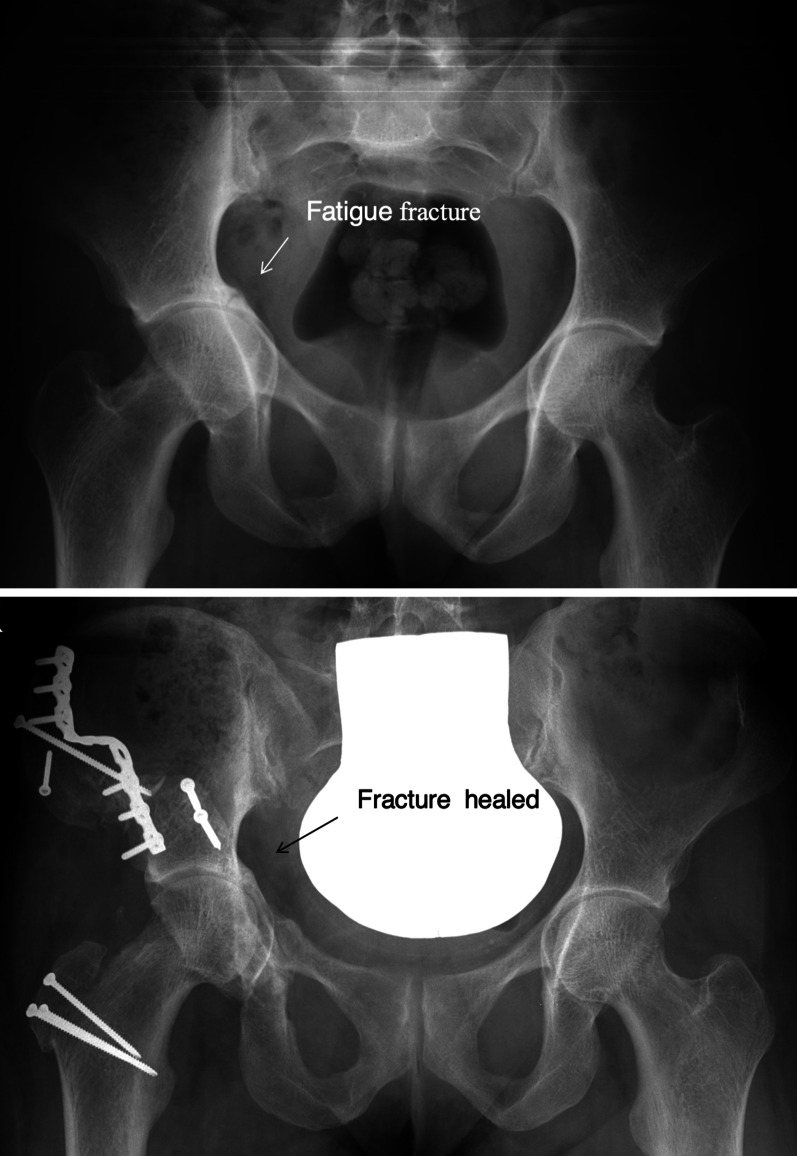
Acetabular protrusio was observed, with a bilateral protrusion in a 21-year-old lady with osteogenesis imperfecta. Constant pain on the right side while the left side was pain-free during most daily activities. Patient refused to get THR. On the top, preoperative radiography showing fatigue fracture through the bottom of the joint (white arrow). b Result 1 year after excessive rim trimming and PAO decovering the head. Fatigue fracture healed (black arrow) with substantially reduced pain
